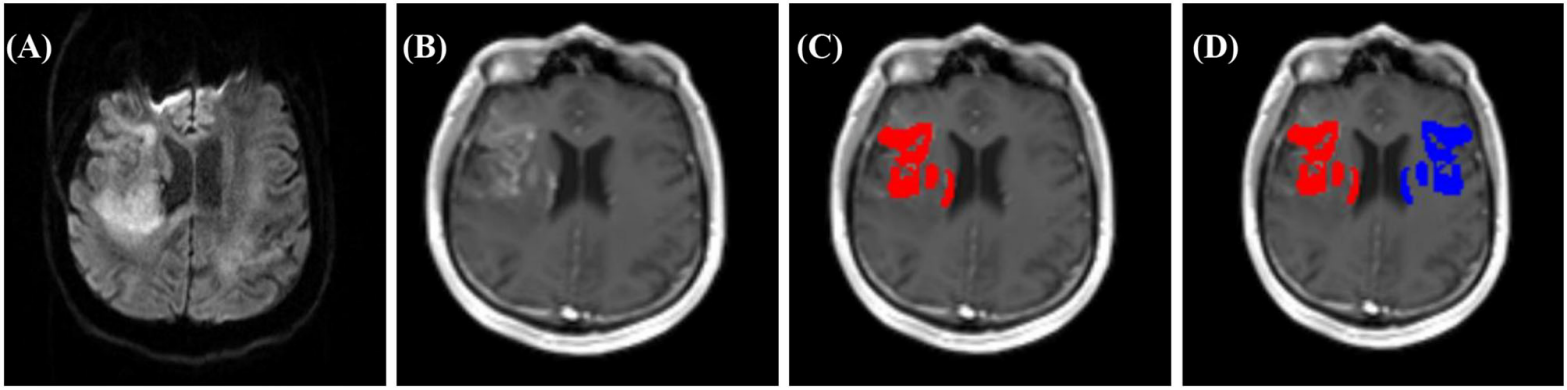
Example of BBBP assessment in a patient with subacute right middle cerebral artery infarction. (A) DWI b1000 for the detection of the infarcted area, (B–D) T1 post-CA sequence: (B) with contrast-agent enhancement (CE), (C) delineation of CE within ischemic lesion (CE-ROI red), with (D) corresponding mirrored ROI (blue).
